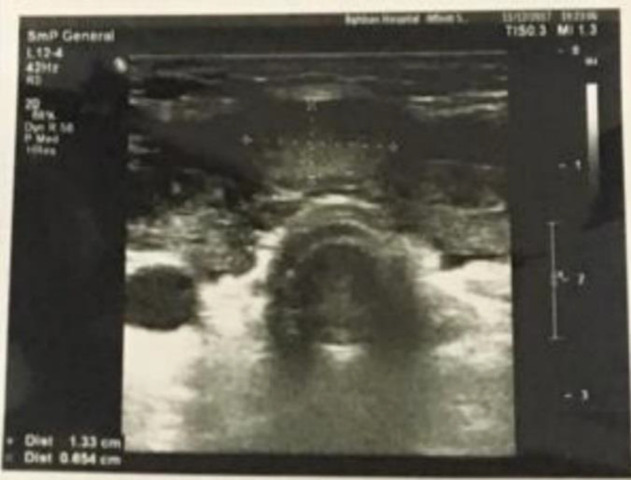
The first thyroid sonography
